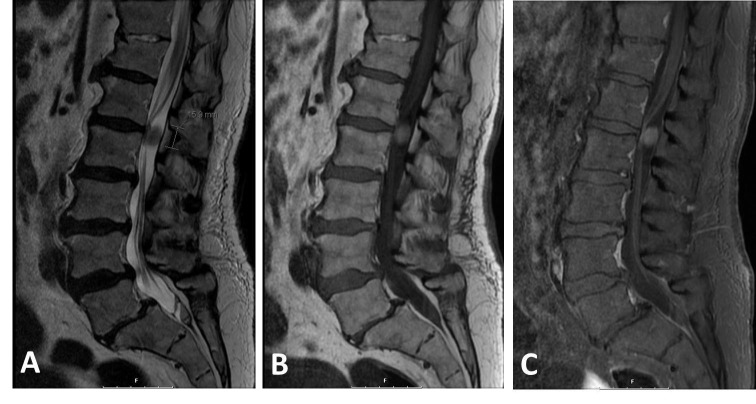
Magnetic resonance imaging (MRI) of spinal schwannoma A: Sagittal T2-weighted sequence showing an intradural extramedullary lesion that is well-circumscribed and has low signal intensity. B: The lesion has a high signal intensity on the T1-weighted sequence and (C) mildly enhances to contrast.
